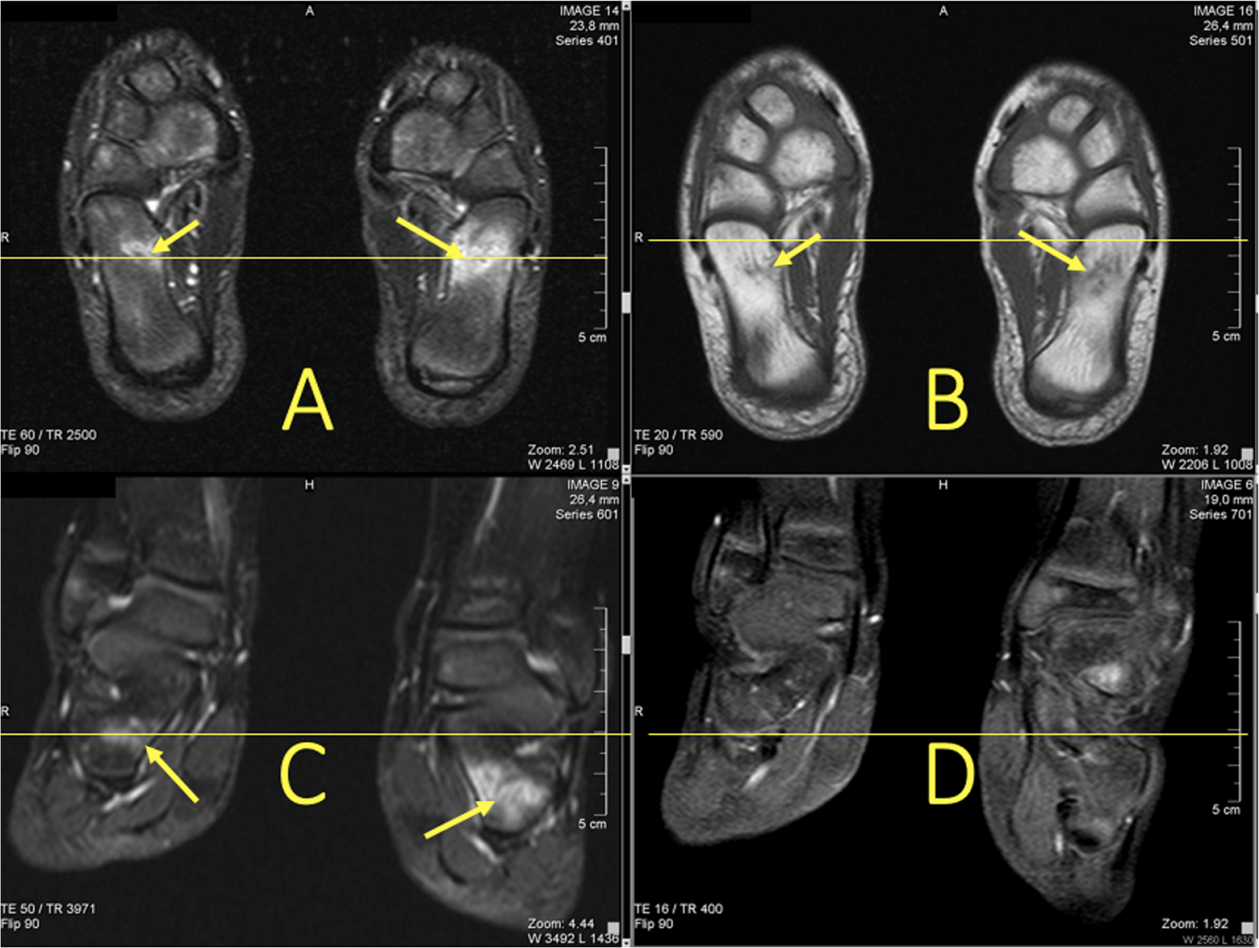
A 7-year-old girl with chronic pain in both feet. MRI shows a developmental variation in the bone marrow signal in the calcaneus (arrows)
